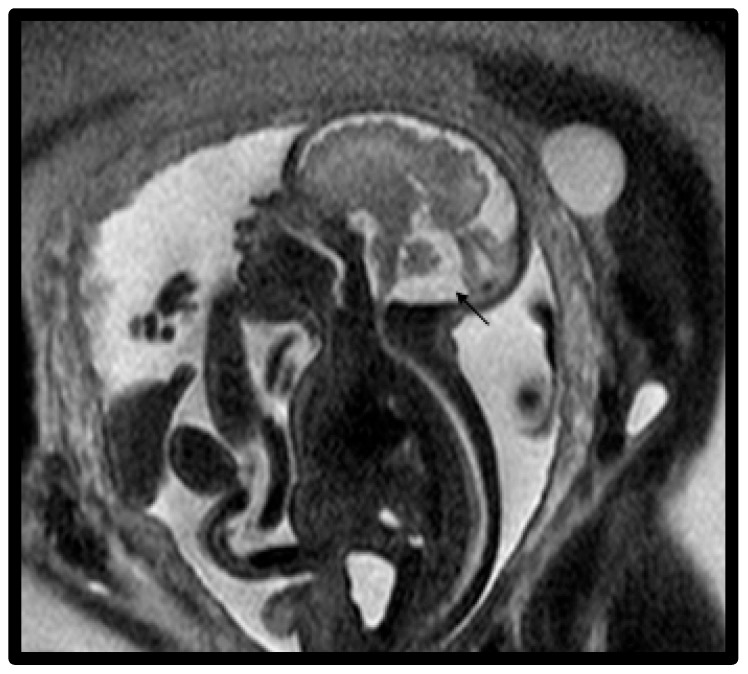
Fetal MRI image of a fetus at 32 gestational weeks with an enlarged cisterna magna. The midline T2-weighted sagittal image shows an enlarged cisterna magna (1.2–1.4 cm) and increased CSF subarachnoid spaces overlying the cerebellum (black arrow). The fourth ventricle did not communicate with the cisterna magna and there was a normally inserted torcula.
